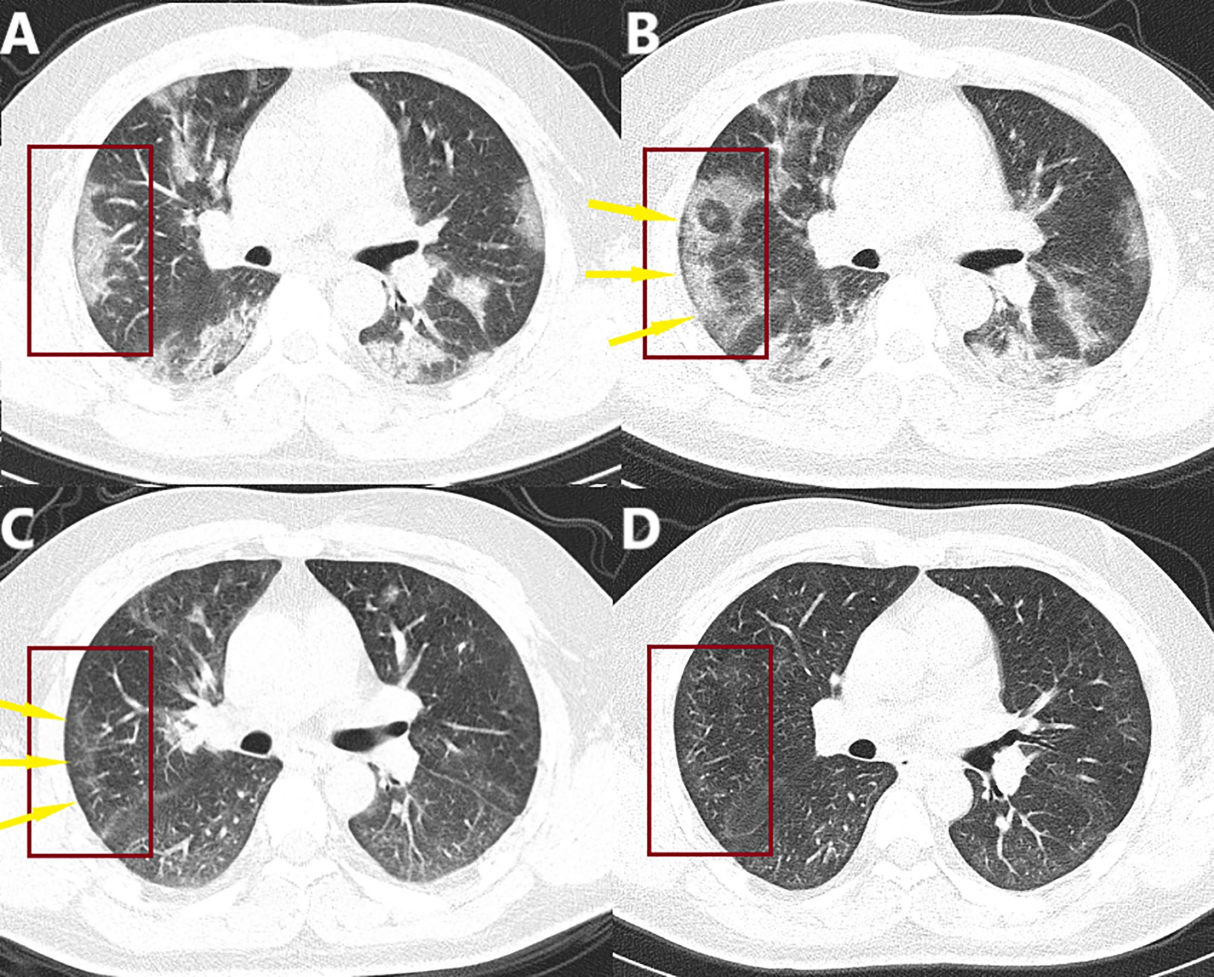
A 34-year-old male who presented with fever for 6 days and cough for 2 days. A. January 21, 2020. The patient’s initial CT scan showed multifocal subpleural conical GGO, locally with consolidation. The patient was diagnosed as severe by chest CT with the semiquantitative score of 25. B. January 26, 2020. The range of lesions (red frame) was enlarged but the density decreased, with a subpleural thin curvilinear lucency (yellow arrow) paralleling the pleural surface, indicating that the lesion is beginning to be absorbed. C. February 26, 2020. The patient’s post-discharge CT review confirmed the initial observation was correct; the lesions were markedly reduced, and the subpleural thin curvilinear lucency (yellow arrow) is still visible. D. March 12, 2020. On reexamination, the lesions had reduced further. However, pulmonary hypoventilation caused by lung tissue damage was also seen, shown as an uneven increase in lung tissue density due to increased X-ray attenuation.
